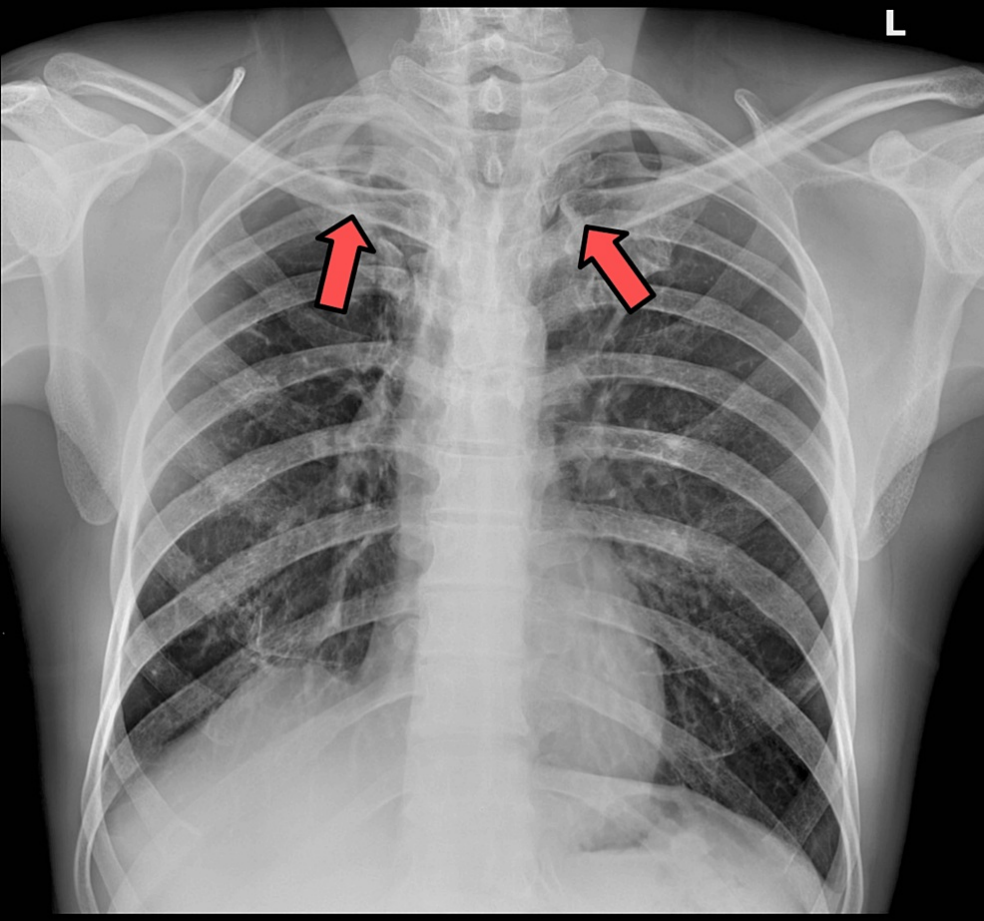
A 50-year-old male with a chest radiograph demonstrating arrows pointing to biapical pleuroparenchymal scarring due to presumed old tuberculosis.
